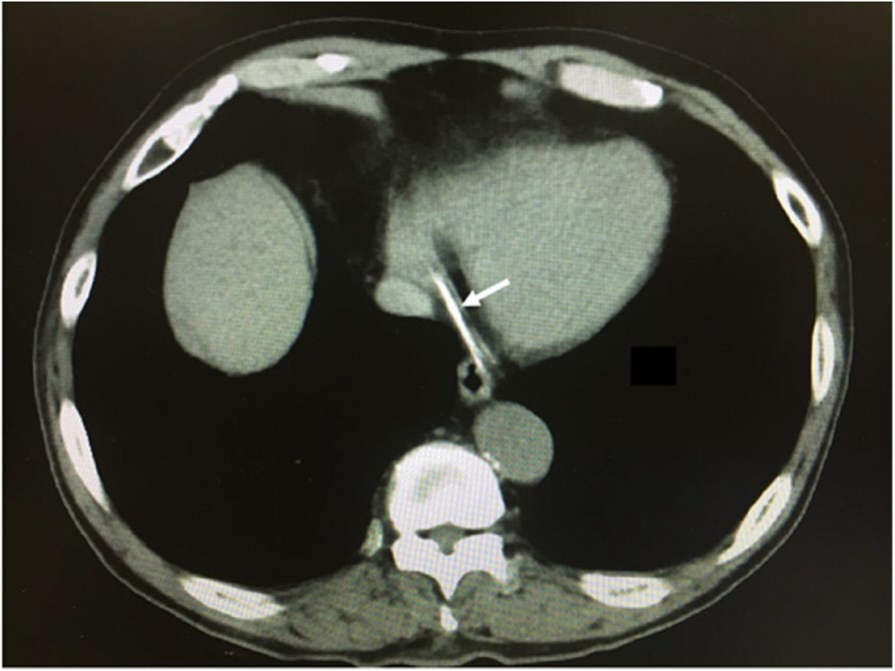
The computed tomography confirmed the presence of a catheter-like foreign body in the coronary sinus and the great cardiac vein (arrows)
